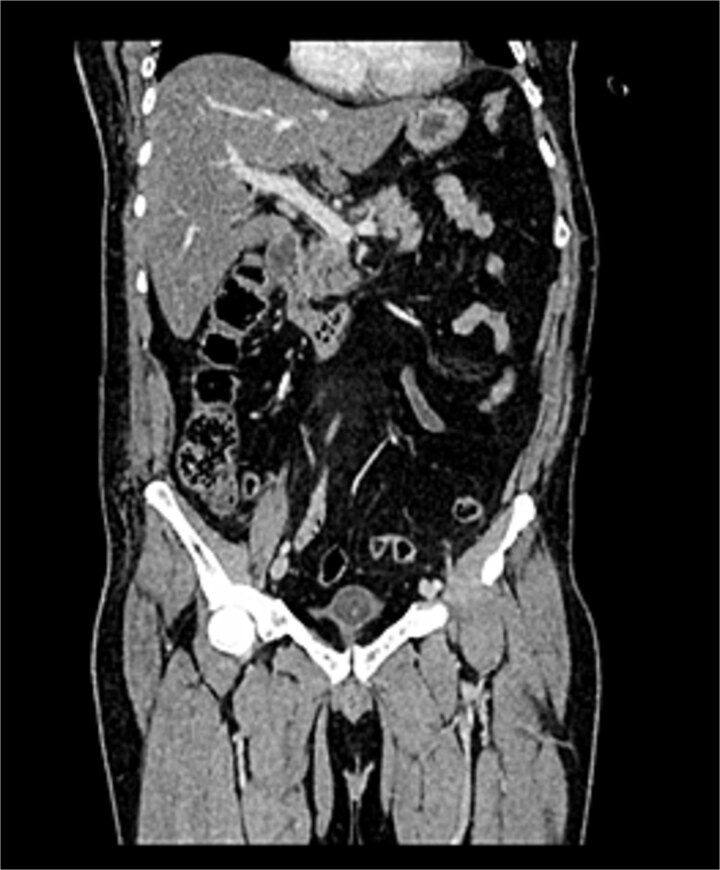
Triphasic abdominal CT, coronal view, demonstrating preserved integrity of the hepatic parenchyma.
